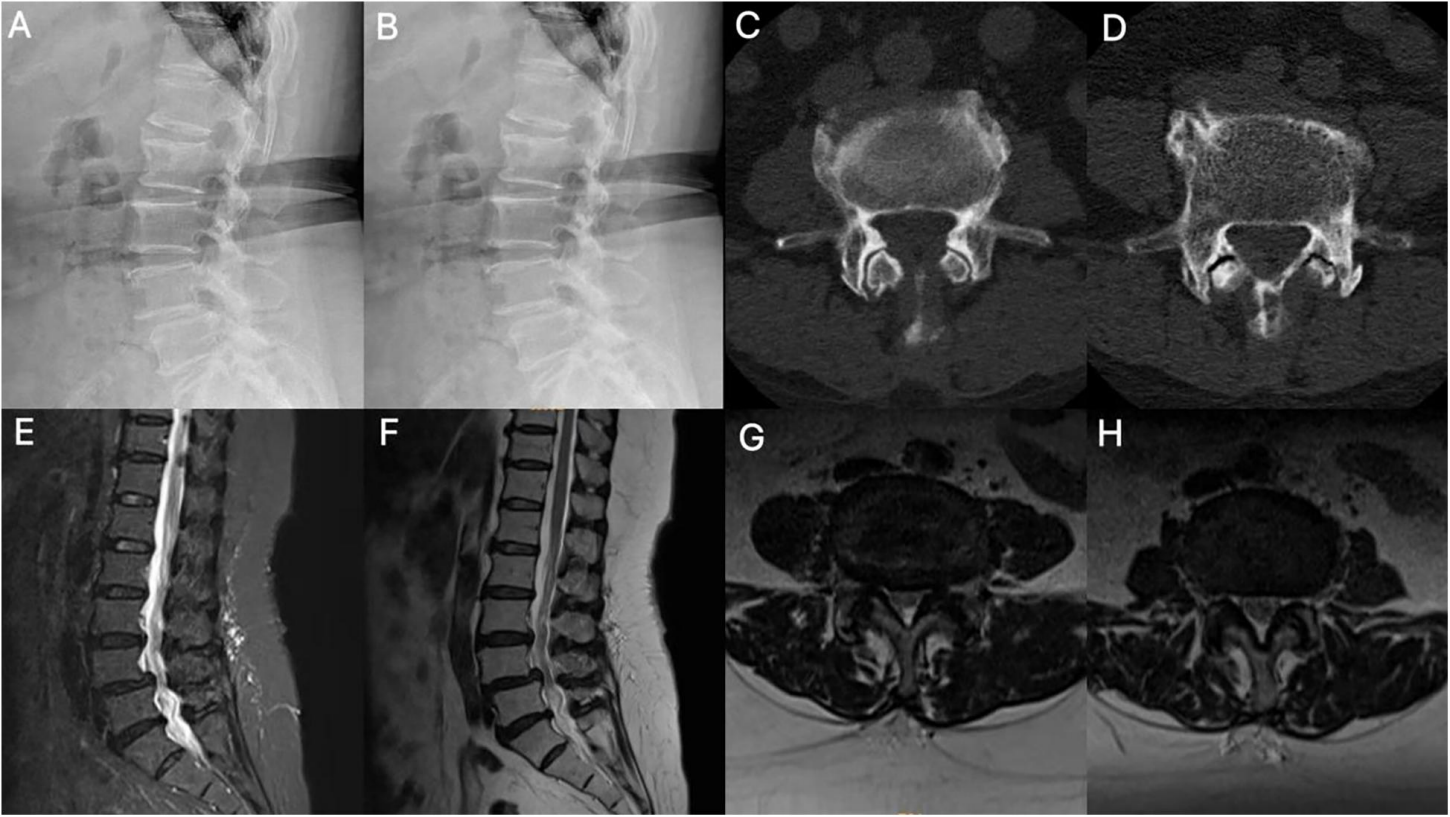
Imaging findings. (A,B) Dynamic lumbar radiographs demonstrate instability with spondylolisthesis. (C,D) Axial CT of the lumbar spine showed segments L3–4 and L4–5 with degenerative changes and lumbar disc herniation. (E-H) MRI shows disc herniation, folded ligamentum flavum causing pincer-type compression of the cauda equina, facet joint hypertrophy, and spinal canal stenosis.
